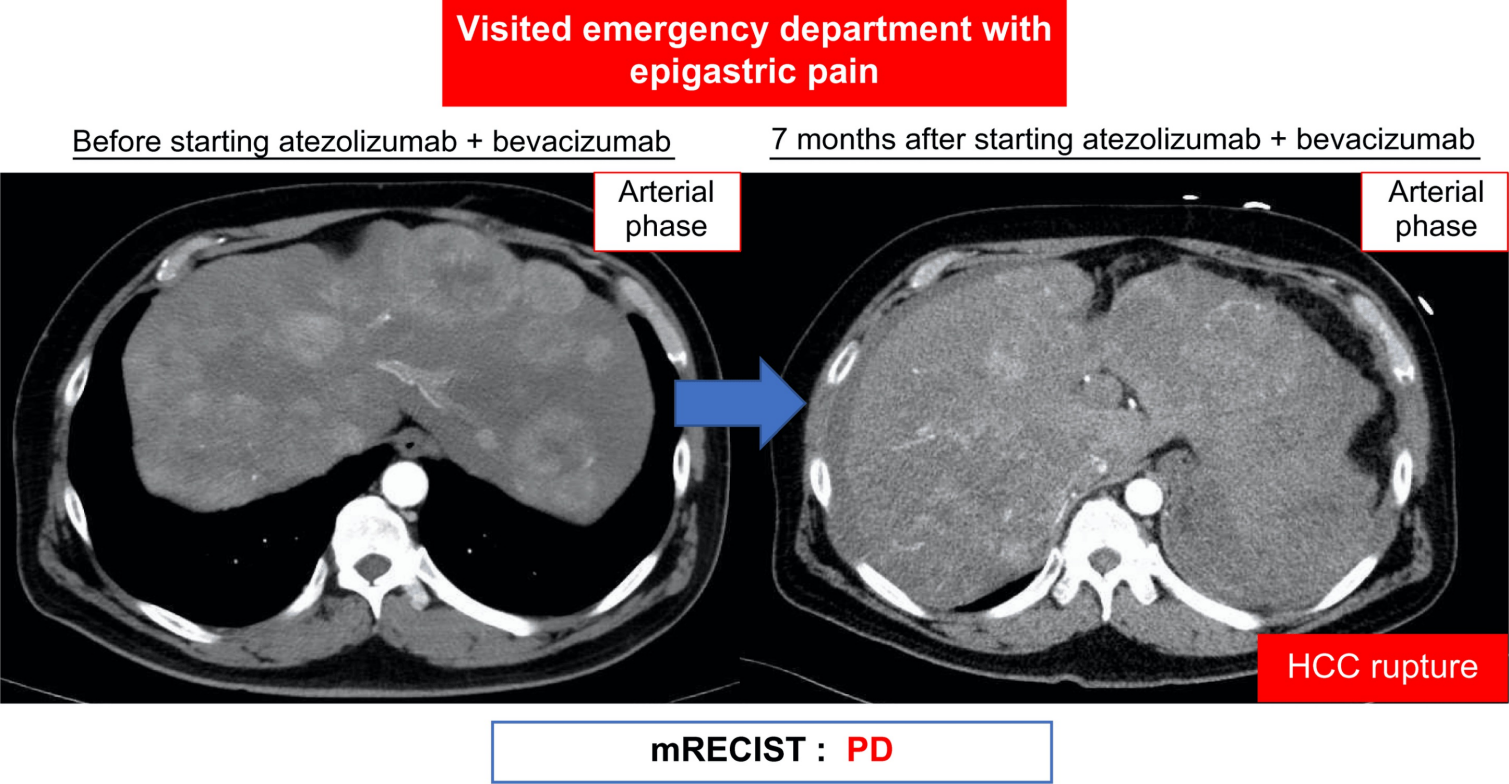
Contrast-enhanced CT tomography revealed hematoma formation around the HCC in the liver S2, leading to a diagnosis of ruptured hepatocellular carcinoma. mRECIST: modified Response Evaluation Criteria in Solid Tumors; PD: progressive disease; HCC: hepatocellular carcinoma
